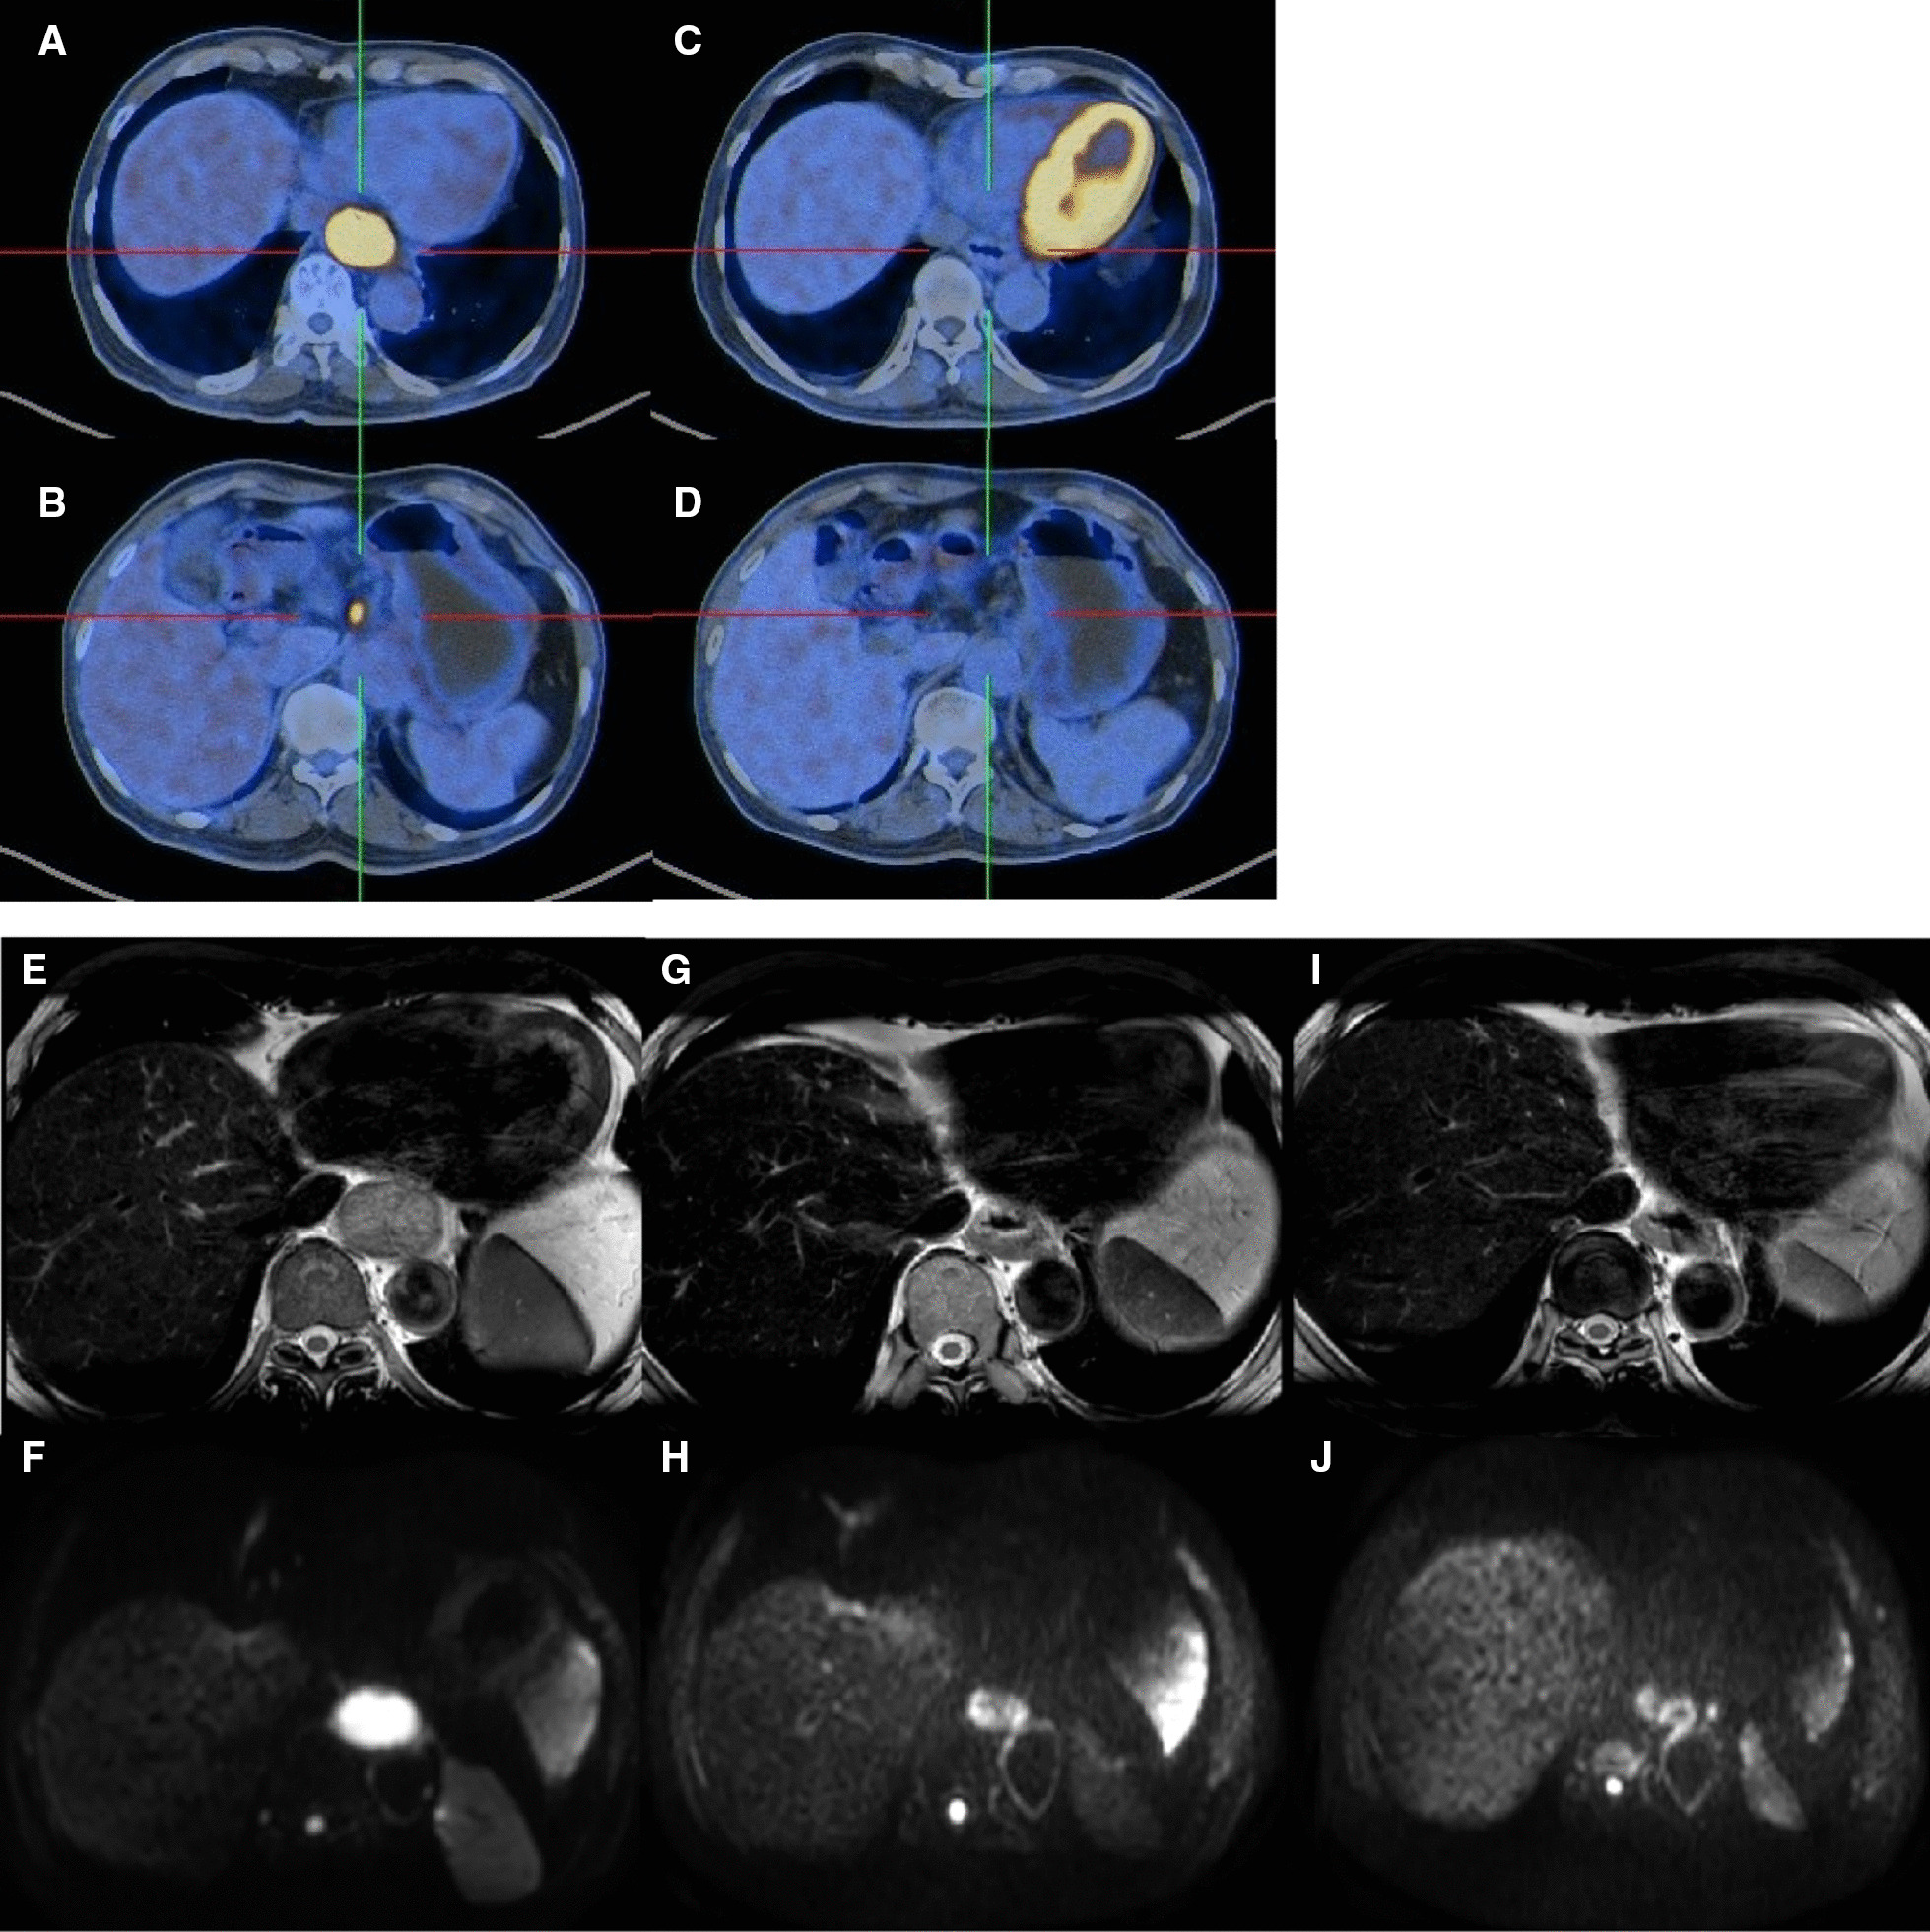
Images from a patient with a cT3N1M0 lower thoracic esophageal squamous cell carcinoma with pathological complete response to neoadjuvant chemoradiotherapy. A, B: PET/CT images before nCRT; C, D: PET/CT images five weeks after nCRT; E, G, I: T2 weighted MRI images, F, H, J: DW-MRI images (b value = 800 s/mm^2^); E, F: before nCRT; G, H: 13 fractions during nCRT; I, J: five weeks after nCRT. *PET/CT* positron emission tomography/computed tomography; *DW-MRI* diffusion-weighted magnetic resonance imaging
